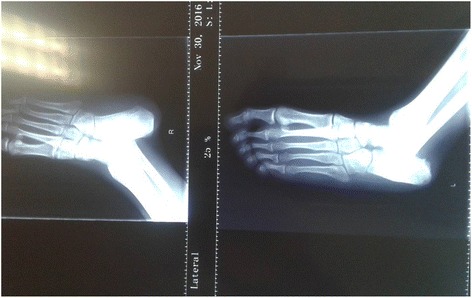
X-ray of the left calcaneus bone showing the bone erosion following osteosarcoma
